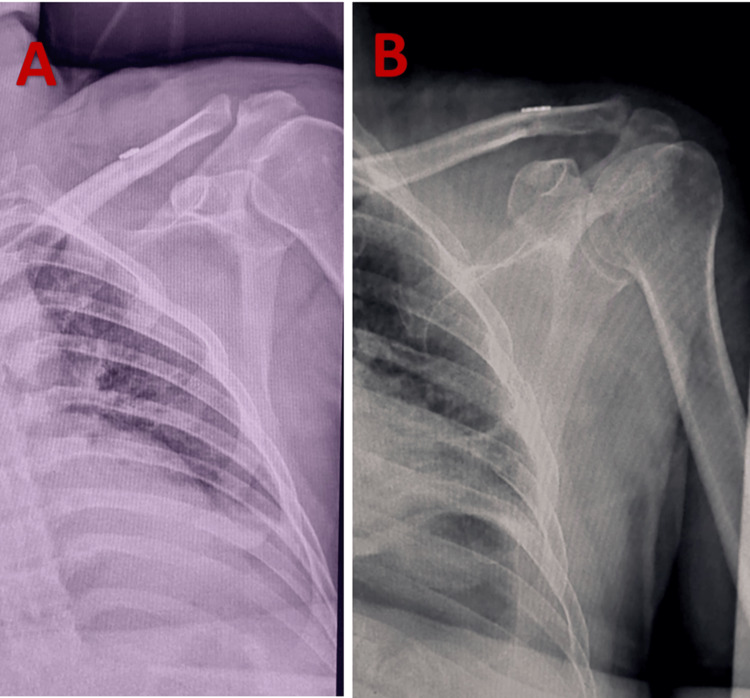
Radiographic follow-up one year after the surgical procedure showing the anatomical reduction of the acromioclavicular joint dislocation (A) Case 1. (B) Case 2.
